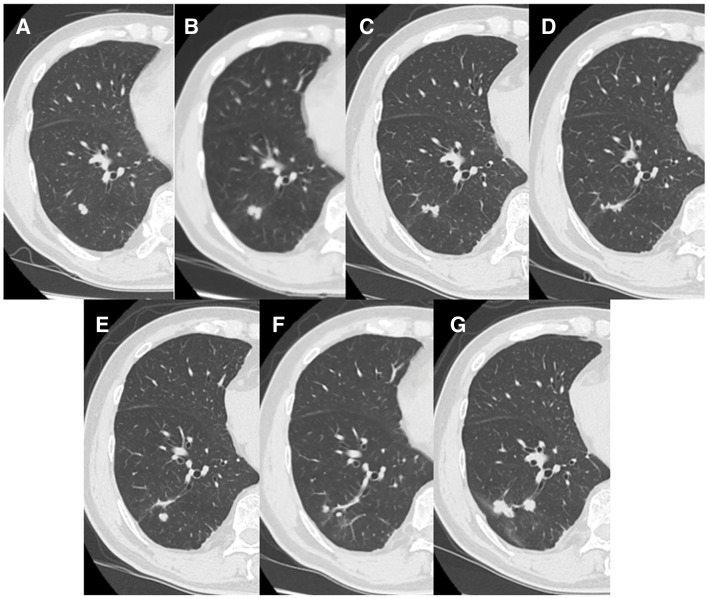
Chest computed tomography findings over time. At the time of initial detection, a snowball-shaped 1.1 × 0.7 cm nodule was seen in S10 of the right lower lobe (A). At the 11-month follow-up, it had mildly enlarged (B); however, it progressively decreased in size on the scans 4 months (C) and 9 months later (D). On the 25-month scan, its size had remained unchanged, but a new small nodule appeared in its vicinity (E). A year later, it had decreased in size again (F). At 49 months, it had enlarged to 3.2 × 0.8 cm (G).
